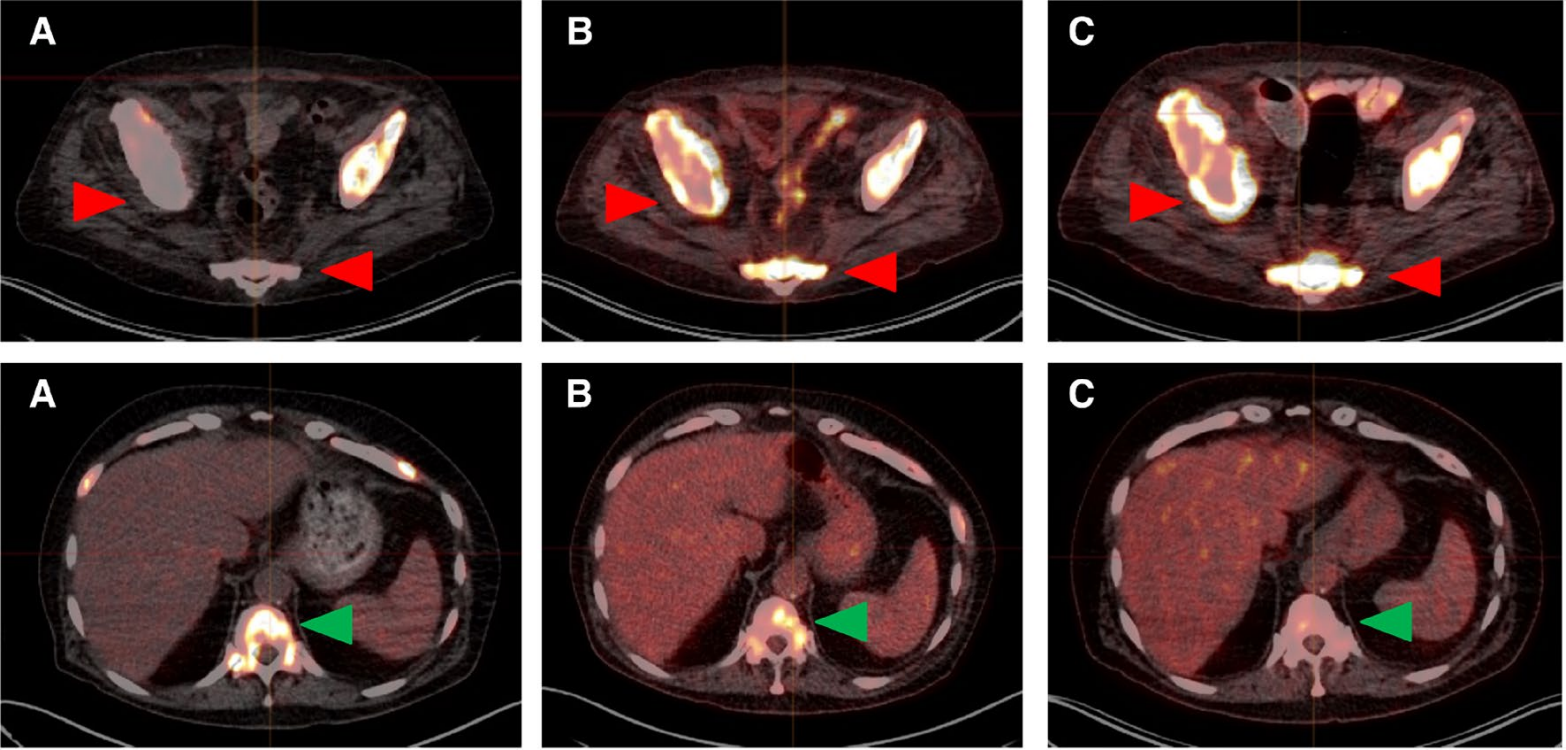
Representative transverse slice images showing lesion-based progression (first row) and partial response (second row) to 2 cycles of [^177^Lu]Lu-PSMA-617 RLT: (A) baseline [^68^Ga]Ga-PSMA-11 PET/CT, (B) baseline [^18^F]FDG PET/CT, and (C) follow-up [^18^F]FDG PET/CT. First row, red arrows: progressing bone metastases. These lesions had faint [^68^Ga]Ga-PSMA-11 uptake (SUV_max_: 3.47 and 4.00, respectively) and intense [^18^F]FDG uptake (SUV_max_: 11.17 and 8.67, respectively) in the baseline scans (FPQ: 3.22 and 2.17, respectively), and increased [^18^F]FDG uptake (SUV_max_ 16.87 and 12.37, respectively; 51% higher and 43% higher, respectively, than at baseline) in the follow-up scan. Second row, green arrows: bone metastasis showing partial response. The lesion, which had intense [^68^Ga]Ga-PSMA-11 uptake (SUV_max_: 22.74) and moderate [^18^F]FDG uptake (SUV_max_: 6.60) in the respective baseline scans (FPQ: 0.29), had decreased [^18^F]FDG uptake (SUV_max_ 4.52, 32% lower than at baseline) in the follow-up scan.
